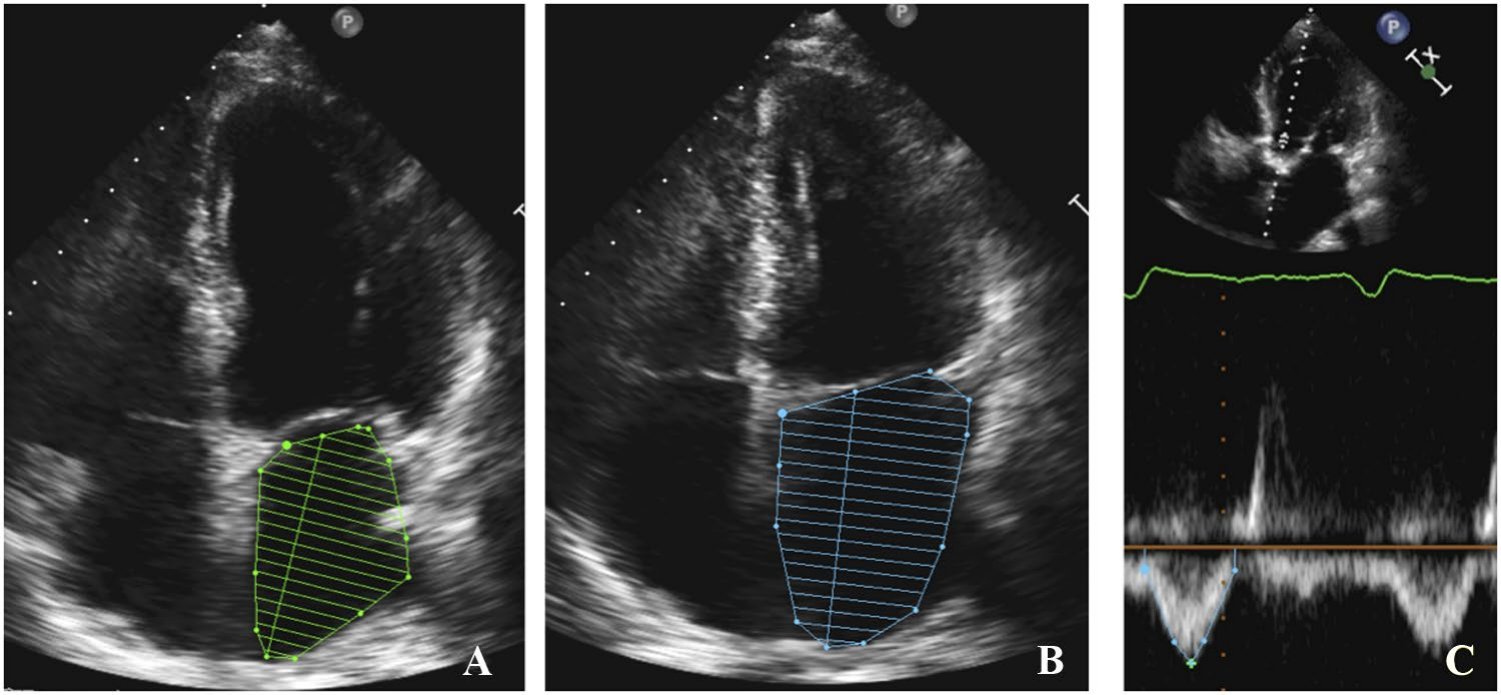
Echocardiographic assessment of the left atrial volume index. Assessment of the left atrial function index using the pre-procedural transthoracic echocardiography. A Measurement of the minimal left atrial volume (LAEDV). B Assessment of the maximal left atrial volume (LAESV). C Calculation of the velocity time integral across the left ventricular outflow tract (LVOT-VTI). *LAEDV* Minimal left atrial volume, *LAESV* maximal left atrial volume, *LVOT-VTI* outflow tract velocity time integral
